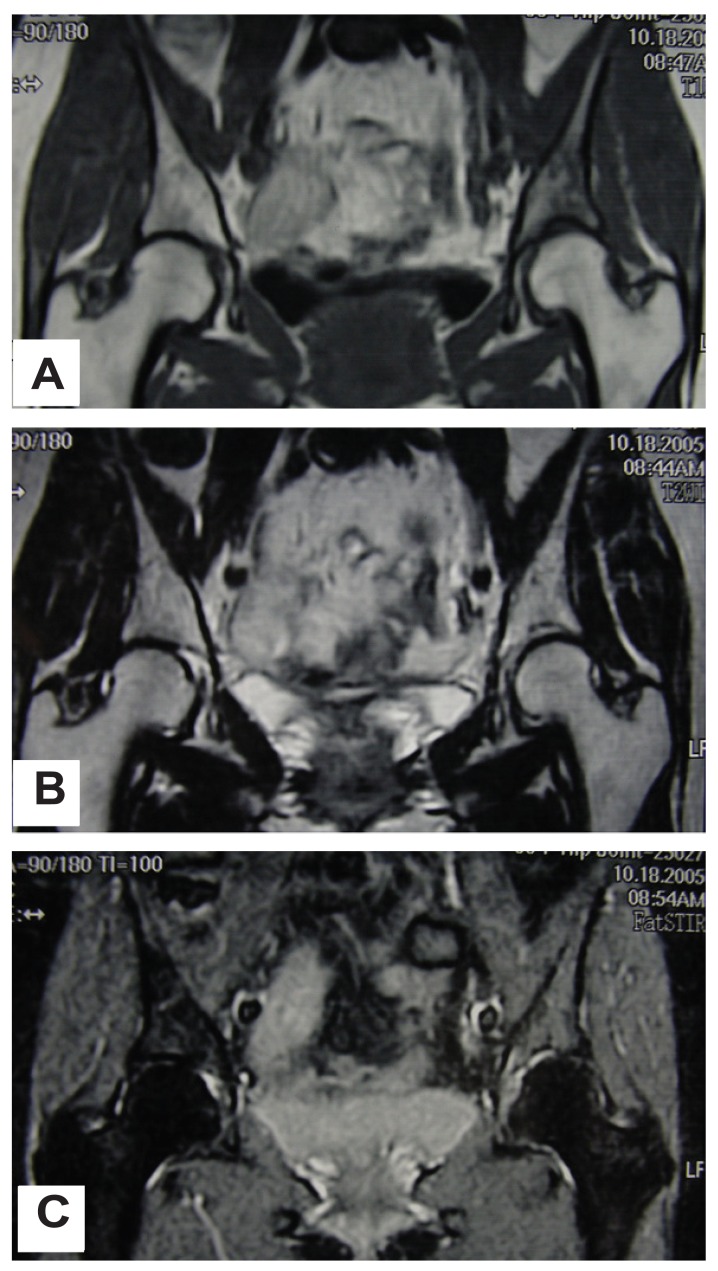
MRI shows diffuse low-signal intensity on T1-weighted image (A) and high-signal intensity on T2-weighted (B) and STIR images (C), suggesting bone marrow edema, associated with the fracture.
